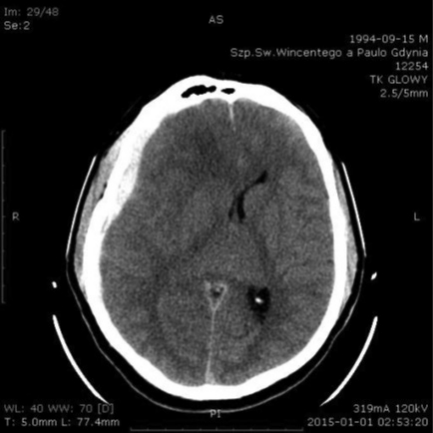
Head CT scan January 1^st^, 2015
